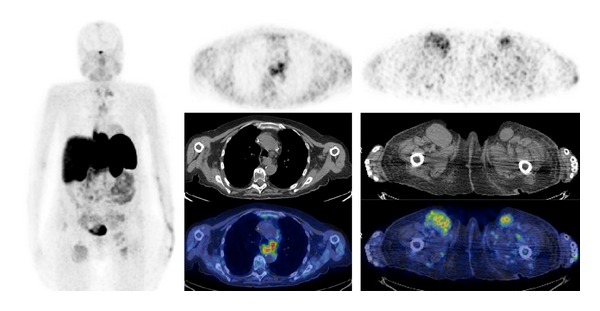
^68^Ga-DOTATATE PET/CT images (MIP, axial) in a patient with non-Hodgkin lymphoma.
